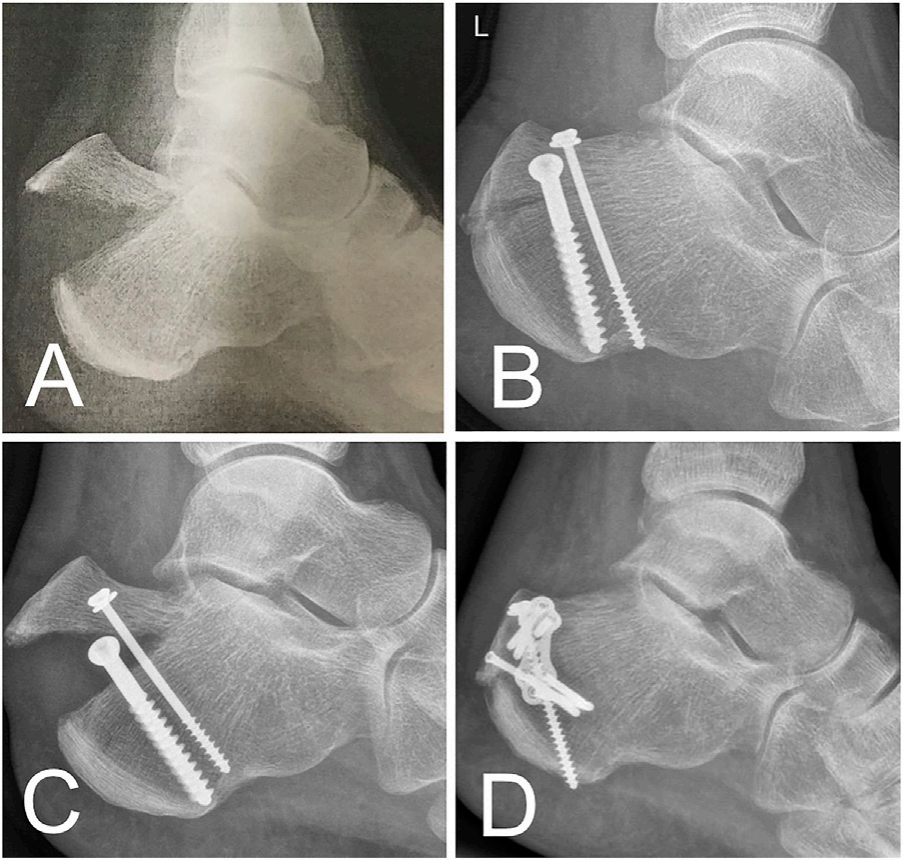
Clinical case. (A) Beak fracture characterized by a solid bone part in a 61-year-old female patient. (B) X-ray after urgent reduction and screw fixation. (C) Early fracture dislocation after mobilization of the patient. (D) Revision performed using a combination of screw and plate osteosynthesis.
